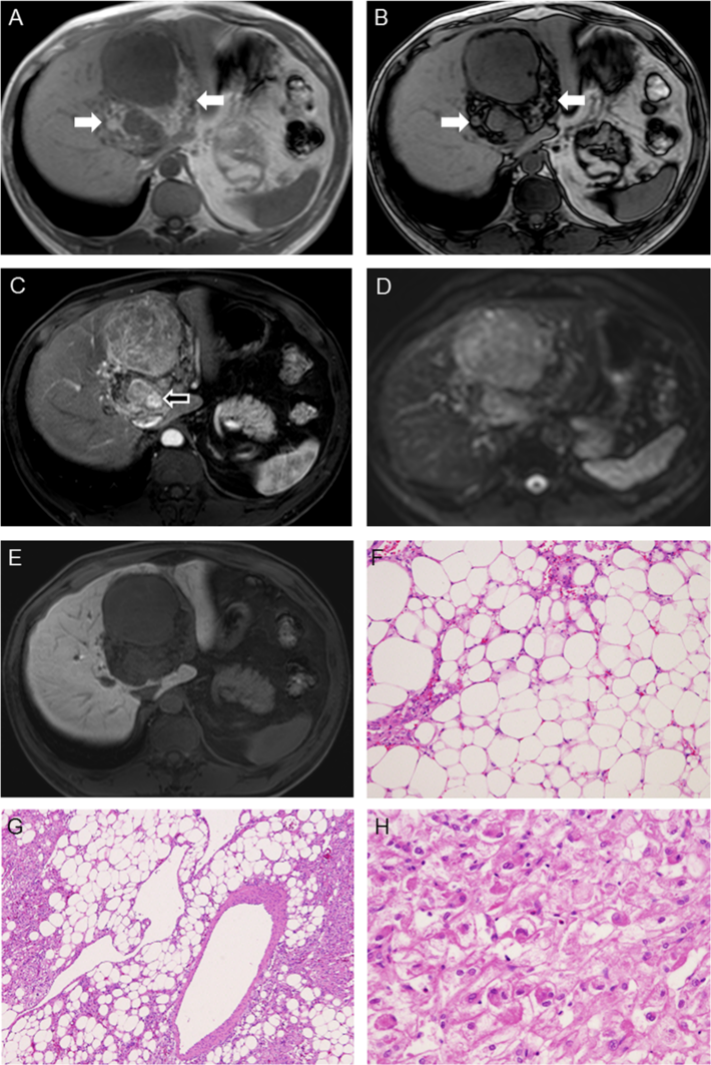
A case of Gd-EOB-DTPA-enhanced MRI findings of huge AML. In the T1W in-phase image (A), the tumor itself is mixed intense in hypointense surroundings. In the in-phase hyperintense signal areas (white arrow), the loose signal in the T1W out-of-phase image (B) is consistent with the fat content (F). In the arterial phase image (C), a minor part of the tumor was remarkably enhanced (arrow) and this part is consistent with meandering blood vessels (G). In DWI, (D) MRI displays an obvious hyperintense signal, whereas in the hepatobiliary phase (E), it shows a homogenous hypointense signal. Major parts of the tumor are consistent with the smooth muscle cell component/components (H), and these lesions were positive for HMB-45 and Melan-A.
